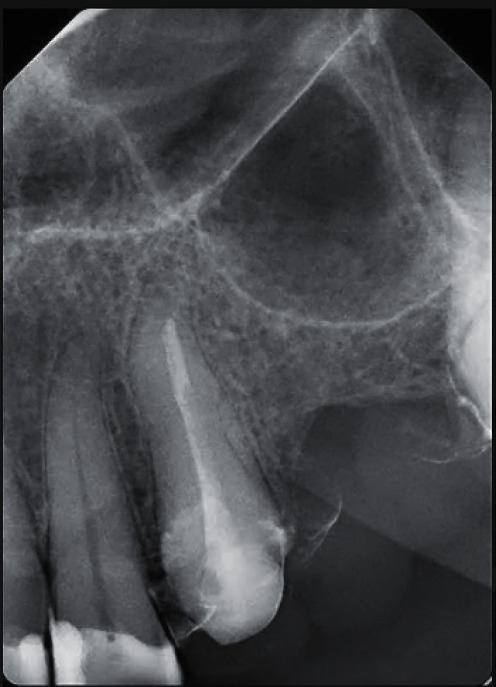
Preoperative radiographic assessment showing tooth #23 with permanent core retained with a fiber post and inadequate root canal filling.
